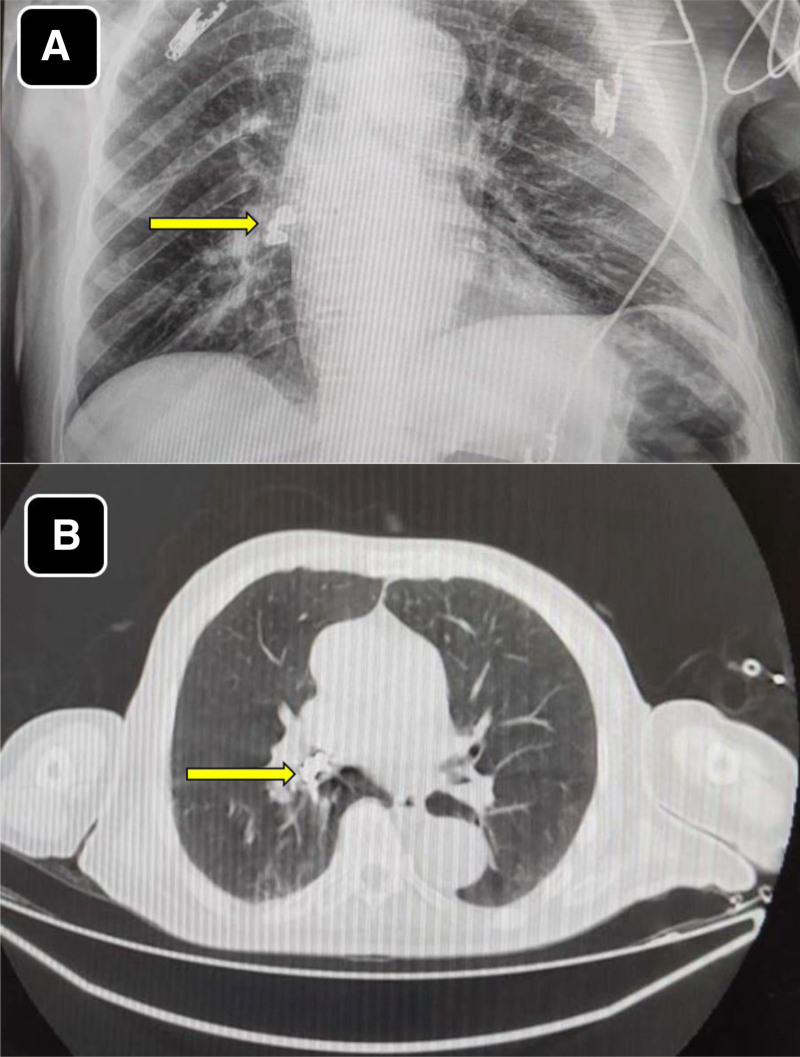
(A) Chest X-ray (AP view) showing the radiopaque tooth in the right-sided bronchus intermedius (yellow arrow). (B) Chest CT scan (lung view) showing the radiopaque tooth residing in the right-sided bronchus intermedius (yellow arrow).
